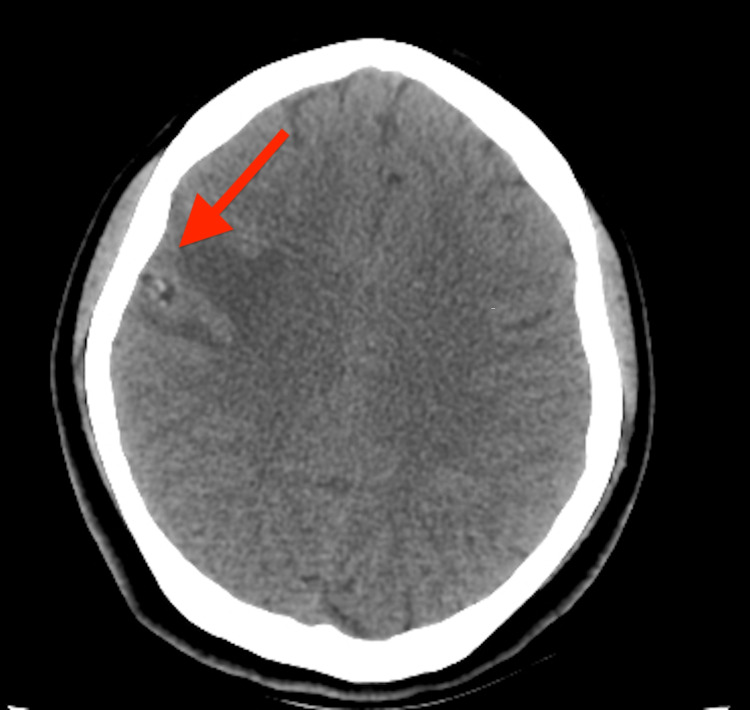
Axial view CT brain showing ring-like hyperdensity with central calcification (red arrow).
